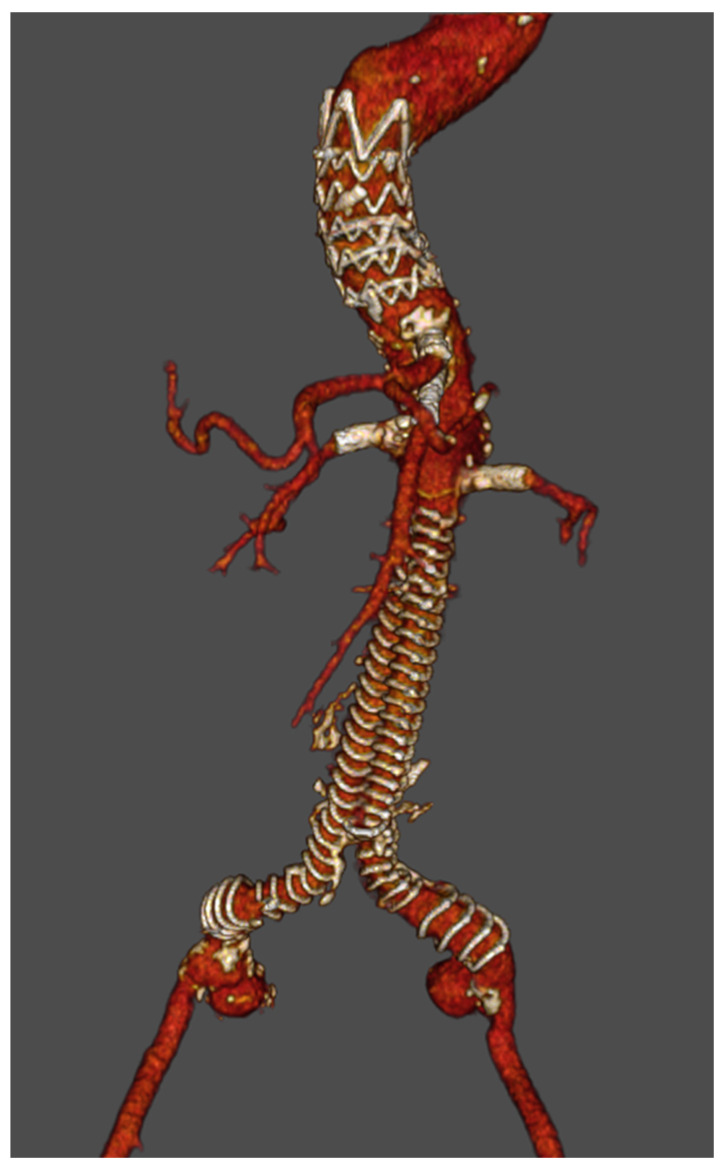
A post-operative CT scan 3D reconstruction showing an exclusion of the abdominal aortic aneurysm.
